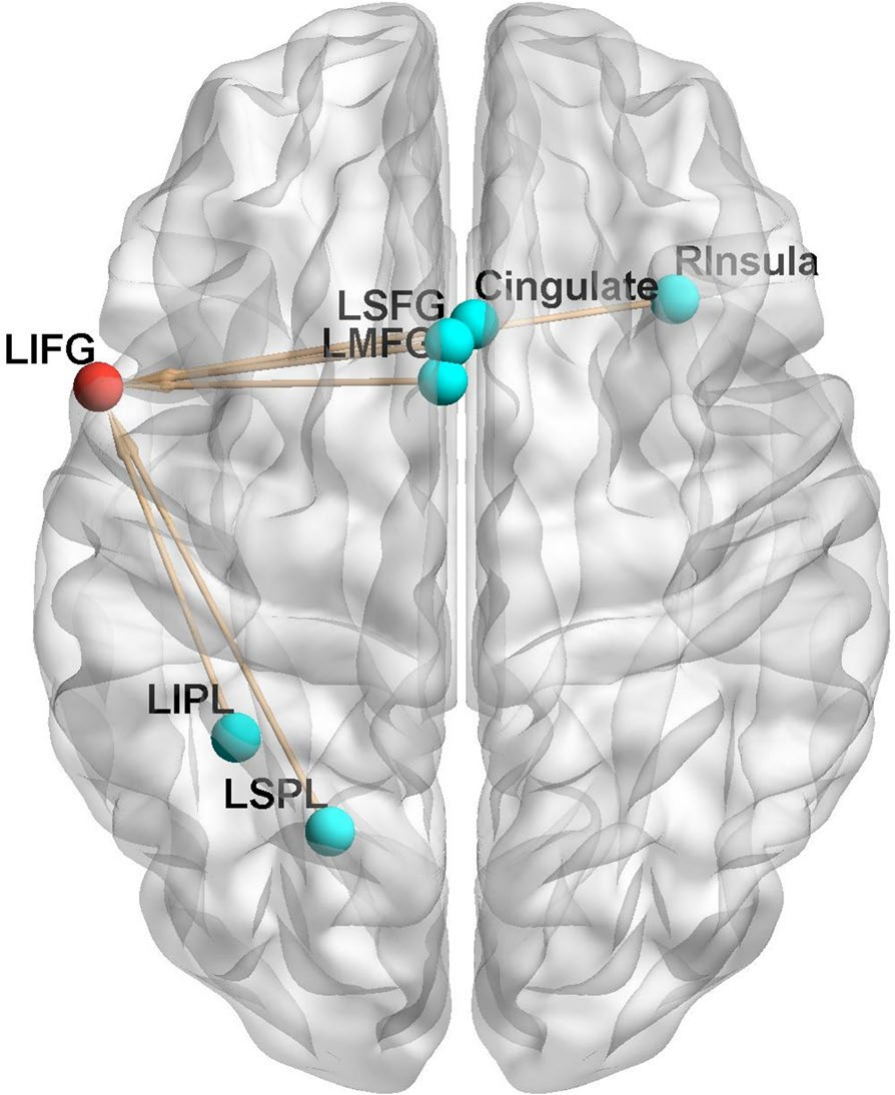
This figure shows the fMACM between the 3 brain regions altered by CT and the 5 executive task-activated regions. Red overlay = left IFG. Light blue overlay =5 executive task-activated ROIs, R Insula (34, 22, 2), Cingulate Gyrus (2, 18, 40), LIPL = L Inf Parietal Lobule (− 36, − 48, 46), LSPL = L Sup Parietal Lobule (− 21, − 63, 51), LSFG = L Sup Frontal Gyrus (− 2, 15, 50), LMFG = L Med Frontal Gyrus (− 3, 9, 50). Arrows are on behalf of co-activations in the fMACM with direction from activation ROI to co-activation overlay
